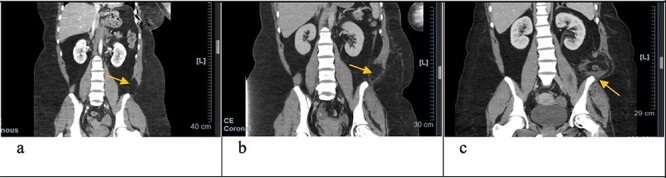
Coronal CT scan with arrows pointing to internal and external oblique defects in June (a), August (b) and September (c); panel (a) shows rupture between internal and external oblique muscles at the attachment point of the quadratus lumborum; panel (b) shows fat stranding, suggesting inflammation of the epiploic appendage, and may be an early sign of delayed traumatic wall hernia; panel (c) shows herniation through the muscular defect, with the descending colon without obstruction.
